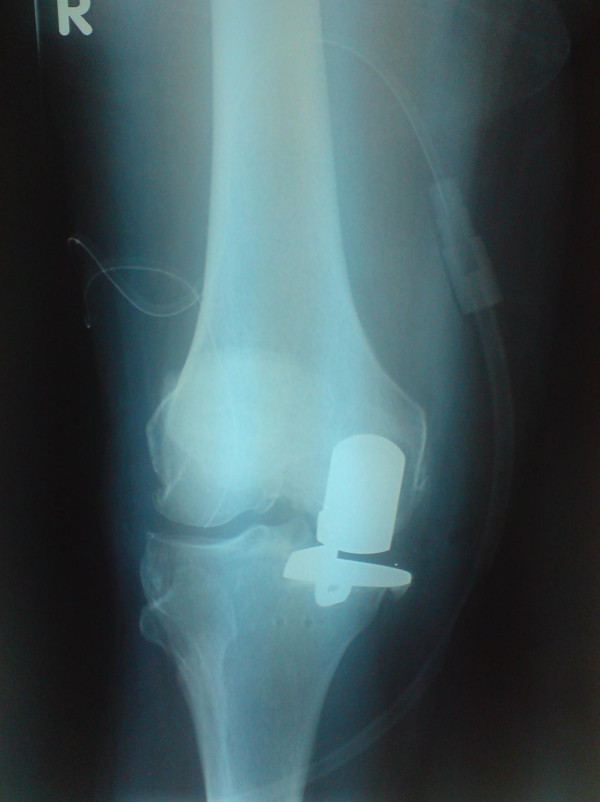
An example of a knee with a poor radiographic score.
